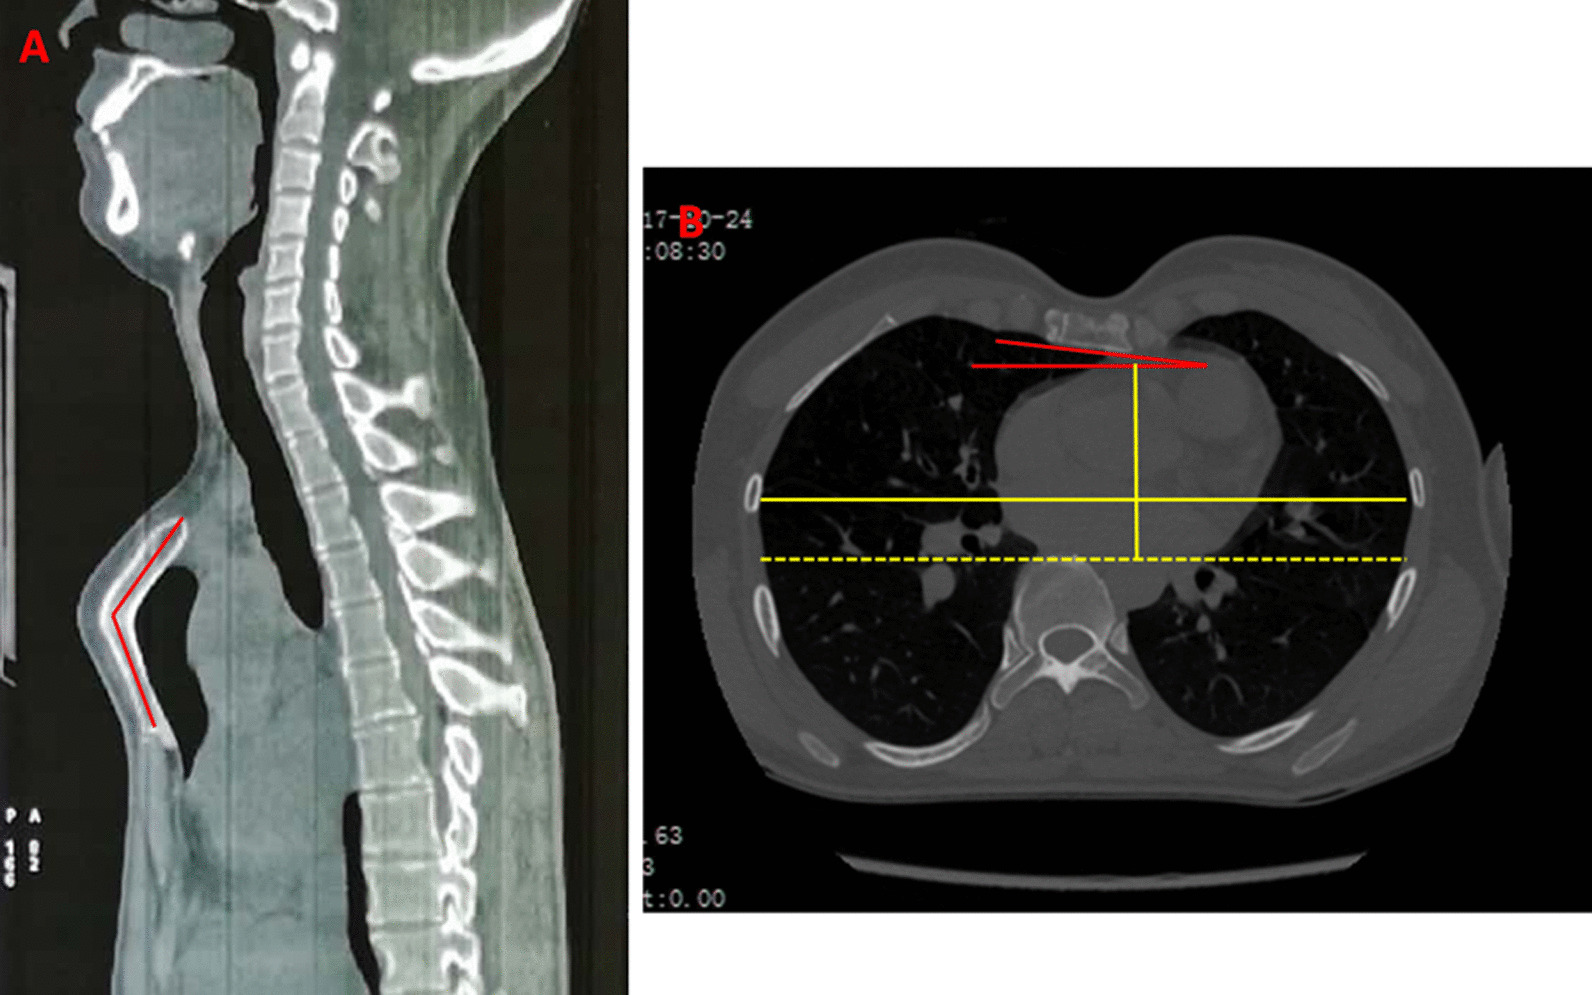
Sagittal CT scan showed the depressed sternum into thoracic cavity. The Louis angle (the angle between the manubrium and the body of the sternum) is 120 degree (A). The coronal CT scan demonstrated central depression of sternum with Haller Index of 3.4 and sternal rotation angle of 7 degree (B)
